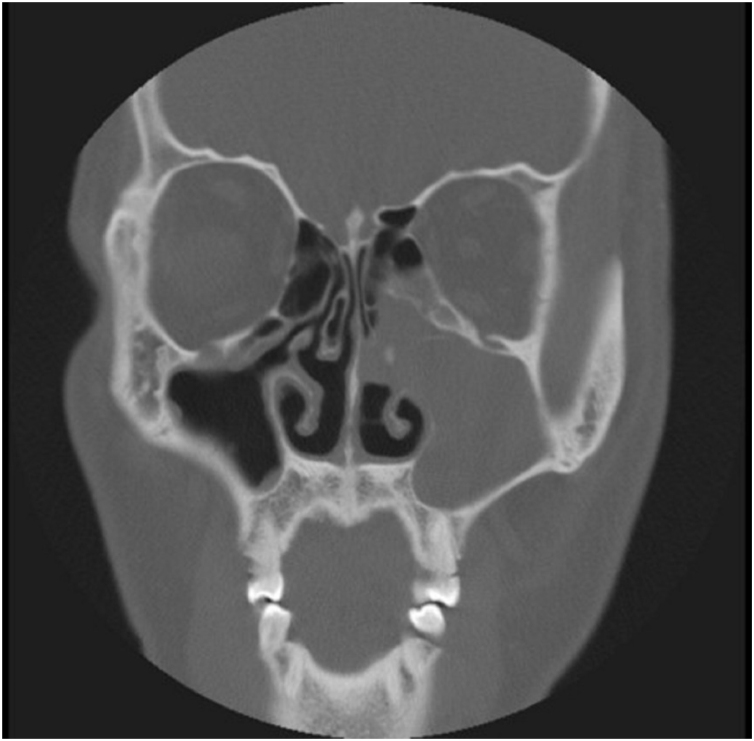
Radiology findings at the first visit, the paranasal sinus computed tomography coronal view revealed a nonhomogeneous soft tissue density in the left maxillary and ethmoid sinuses without bony remodeling.
